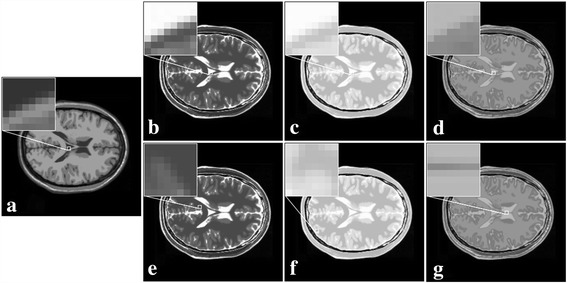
Sub-regions with same or different anatomical structures in synthetic MRI images in (a-g)
